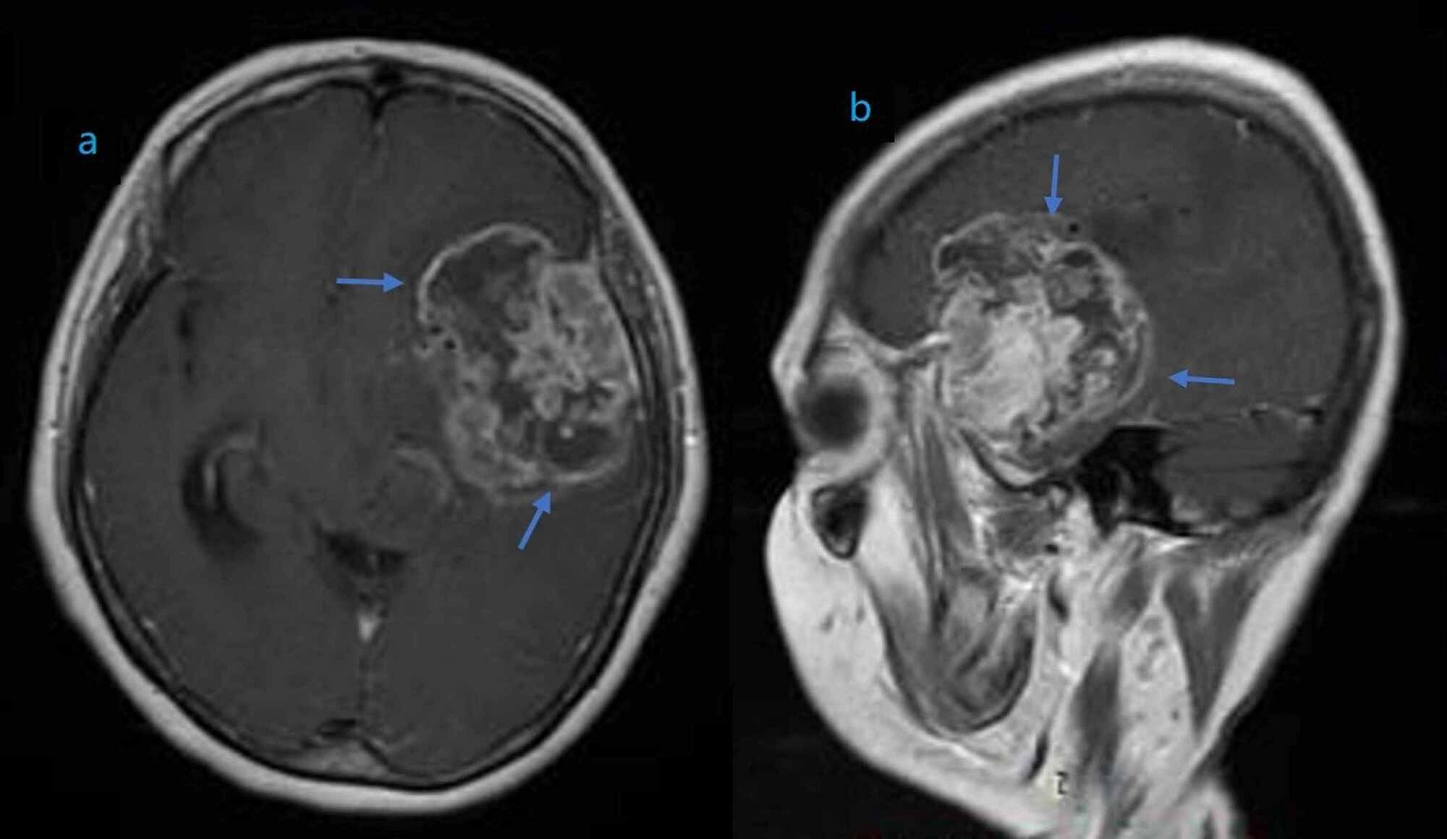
Magnetic resonance imaging (MRI) of the brain showing an enhancing mass of heterogeneous density involving the left temporal lobe in coronal (a) and sagittal (b) planes.
